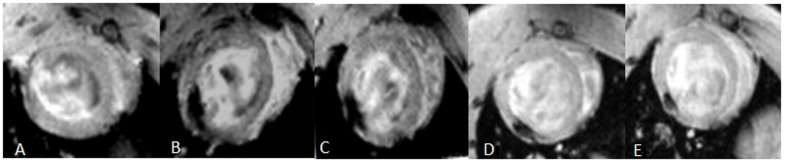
Serial MRI. (A) Control saline-injected heart had no hypointensities. (B) Injection of 1 × 10^6^ SPIO-labeled MSCs 3 days created a wide intramural area of hypointensity (arrows) at the anterior LV wall. (C–E) Positive magnetic signals (arrows) are still visible at 1 w, 2 w, 4 w after MSCs injection respectively, but the signal intensity changed gradually.
